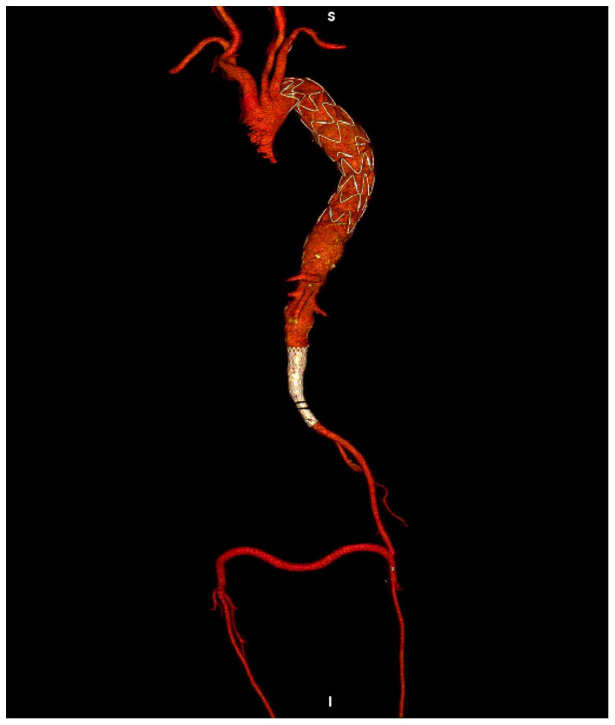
Post-operative CTA demonstrating correct positioning of the stent grafts and regular patency of the bypass.
